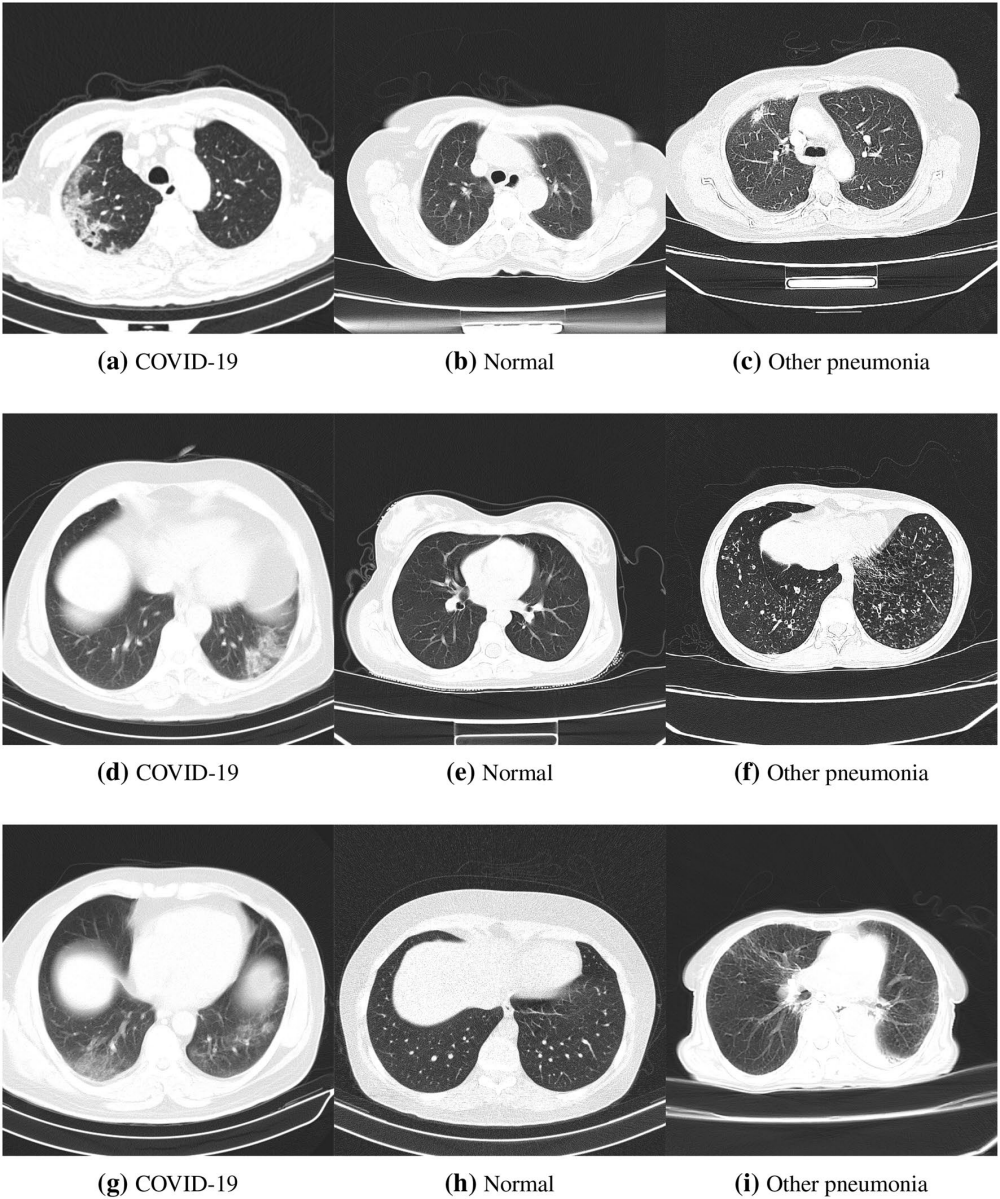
Several different instances of the proposed dataset, i.e., CT-COV19: each column provides several examples of each class presented in the dataset.
